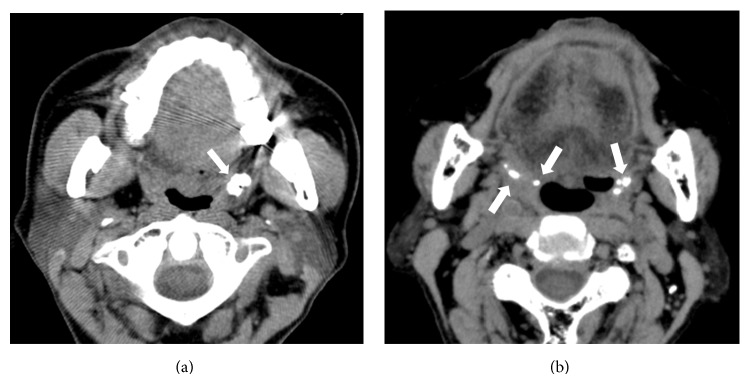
Axial CT images. (a) Single large tonsillolith in the left palatine tonsil (white arrow). (b) Multiple small tonsilloliths involving right and left palatine tonsils (white arrows).
